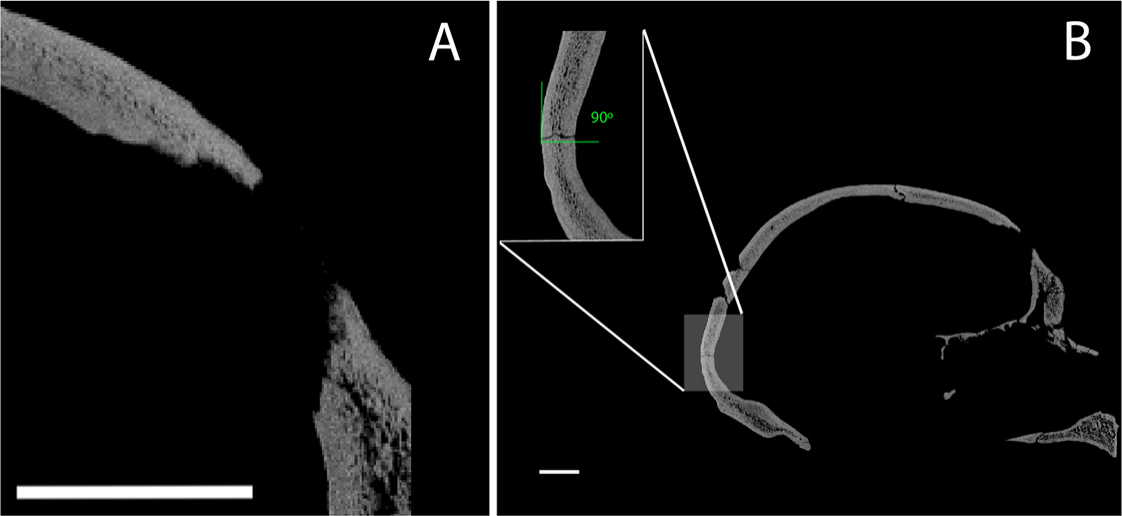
CT cross-sections comparing perimortem and postmortem fractures in the SH crania. (A) Perimortem fracture of the frontal bone in SH Cranium 17; (B) Postmortem fracture in the occipital bone of SH Cranium 17 showing a right angle at the point of breakage. Scale bar = 2 cm.
